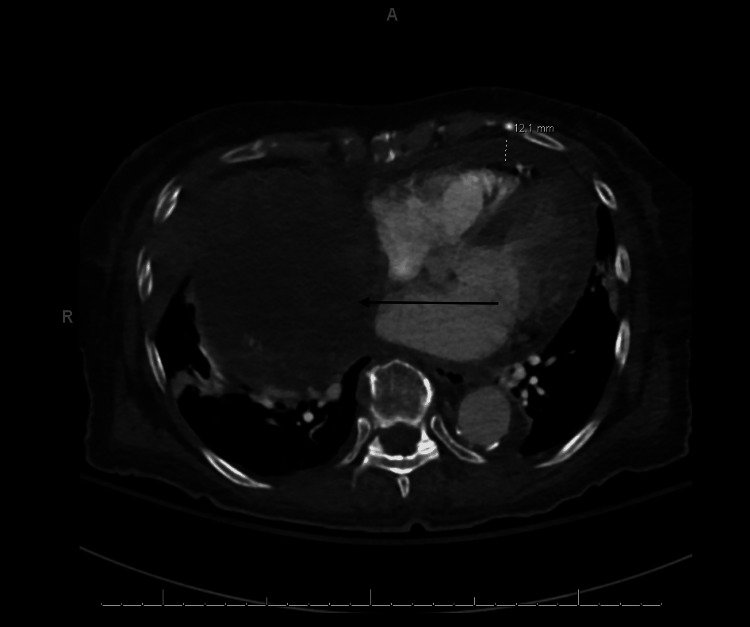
CT pulmonary abdomen pelvis (axial view). This figure shows an axial view of a CT scan of the pulmonary abdomen pelvis, highlighting the superior vena cava (SVC) and right atrium being compressed by a large ovoid, solid-appearing, heterogeneously enhancing mass (indicated by the arrow).
